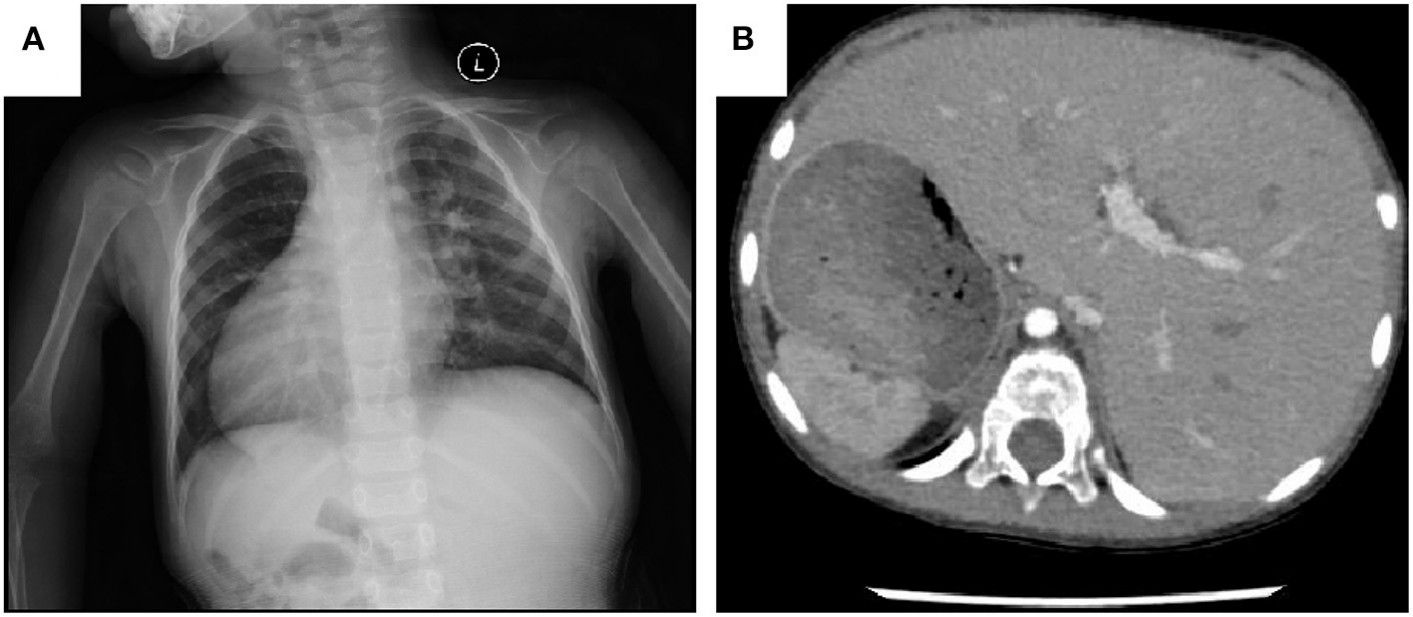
(A) Chest X-ray shows the cardiac apex directed to the right side. (B) CT scanning of the abdomen shows the liver on the left side, the stomach and spleen on the right.
